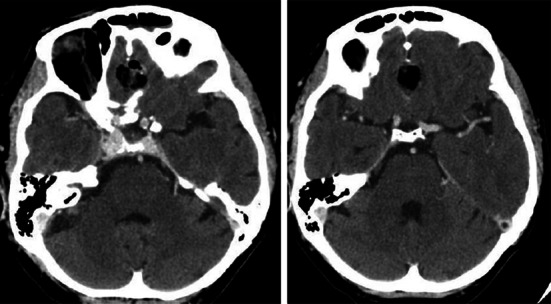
Case 3—follow-up CT scan
